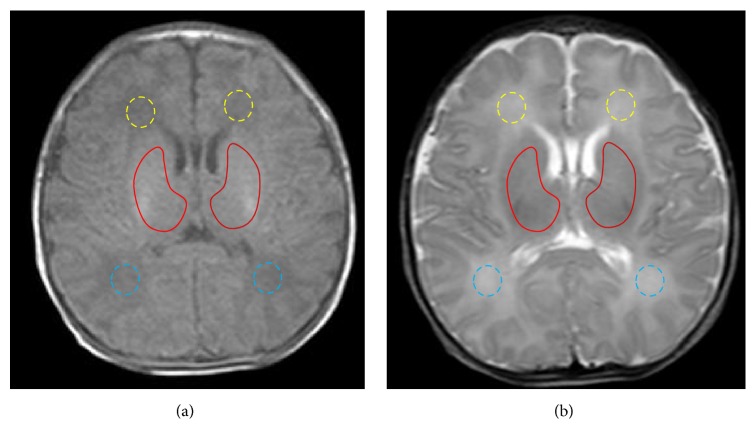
ROIs selection. Images from T1WI (a) and T2WI (b) sequences in the conventional MRI examination are referenced for the selection of ROIs in this study. For all neonates, ROIs are chosen bilaterally in the frontal lobe deep white matter (yellow dotted line), basal ganglia (solid red), and occipital lobe white matter (blue dotted line) bilaterally.
